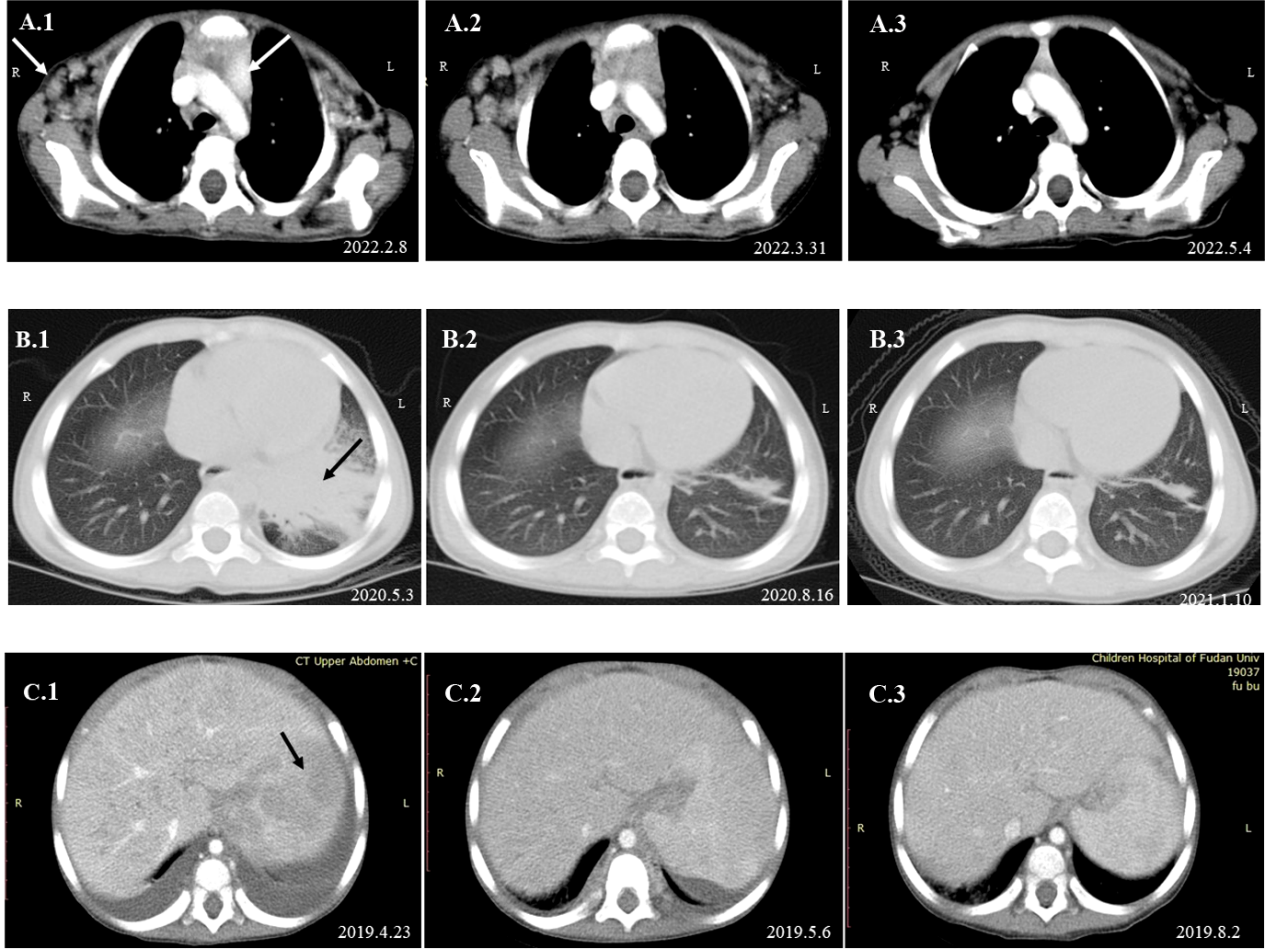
Dynamic changes in imaging examinations of patients during follow-up. Imaging examination of P1 revealed that the axillary and mediastinal lymph nodes were enlarged (A.1). After oral itraconazole and anti-tuberculous therapy for nearly 2 months, chest CT re-examination showed that the axillary and mediastinal lymph nodes were still enlarged (A.2). A lymph node biopsy was performed on April 19, 2022, and mNGS indicated high reads of T. marneffei. Antifungal therapy was adjusted to amphotericin B for 2 weeks, followed by oral itraconazole. One month later, the imaging examination suggested that the lymph nodes were smaller than before (A.3). Chest CT of P3 suggested pneumonia and partial consolidation of the left lung with slight pleural effusion in the acute phase (B.1), and the patient received oral itraconazole antifungal combined with anti-tuberculosis therapy. Chest CT re-examination revealed significant improvement in the lungs after 3 months (B.2) and 8 months (B.3). Abdominal CT of P5 indicated hepatosplenomegaly and multiple abnormal lesions at the beginning (C.1). He received intravenous voriconazole treatment for 25 days and then oral voriconazole. The multiple abnormal lesions improved after treatment for 2 weeks (C.2) and 3.5 months (C.3).
